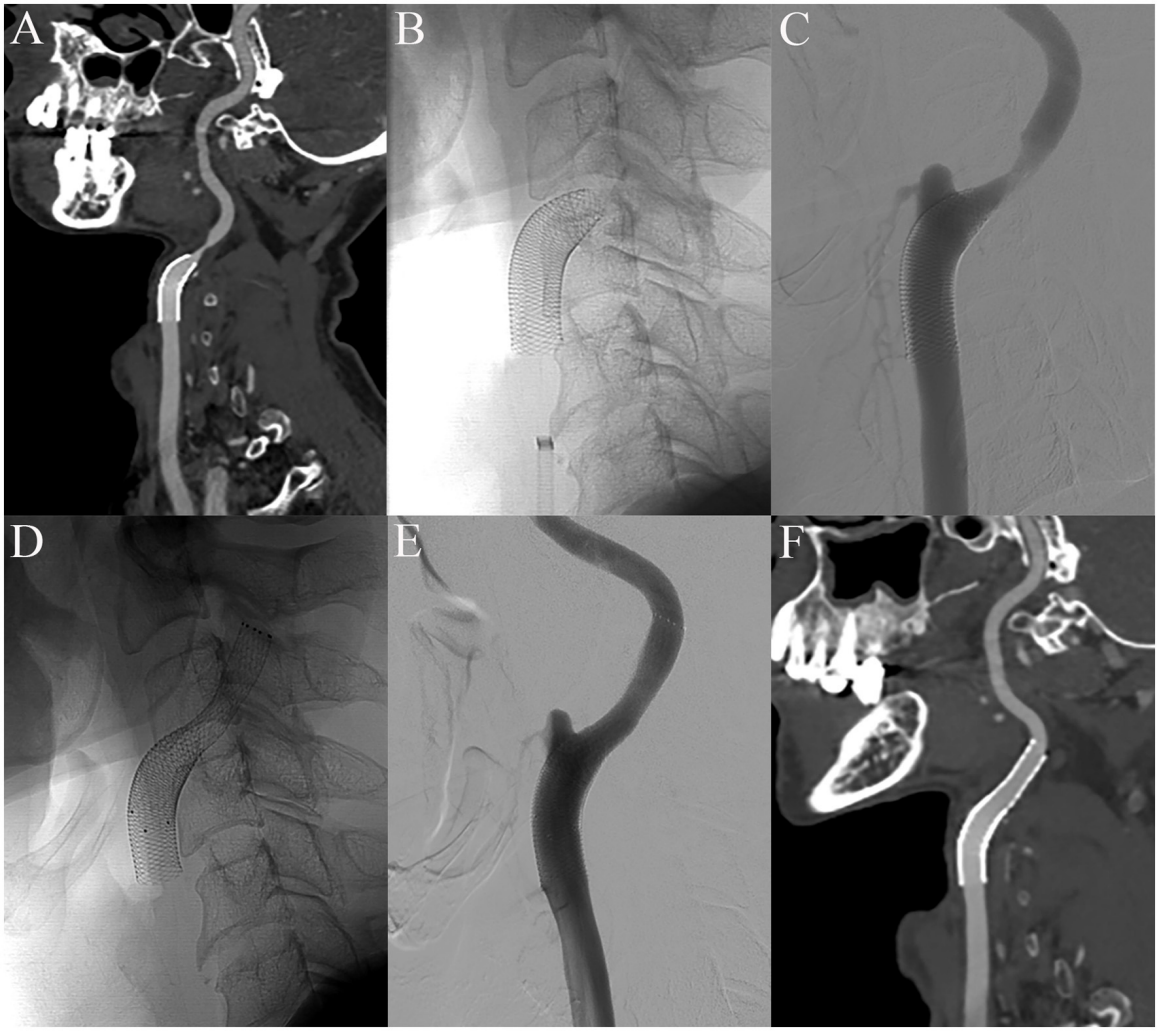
The day after CAS, early shortening of the CAS and distal stenosis are demonstrated in CTA and the cerebral angiogram (A–C). After additional carotid stenting, the final angiography showed successful expansion and implantation of the carotid stent (D, E). There was no shortening and malposition of the CAS 1 month after additional carotid stenting (F).
